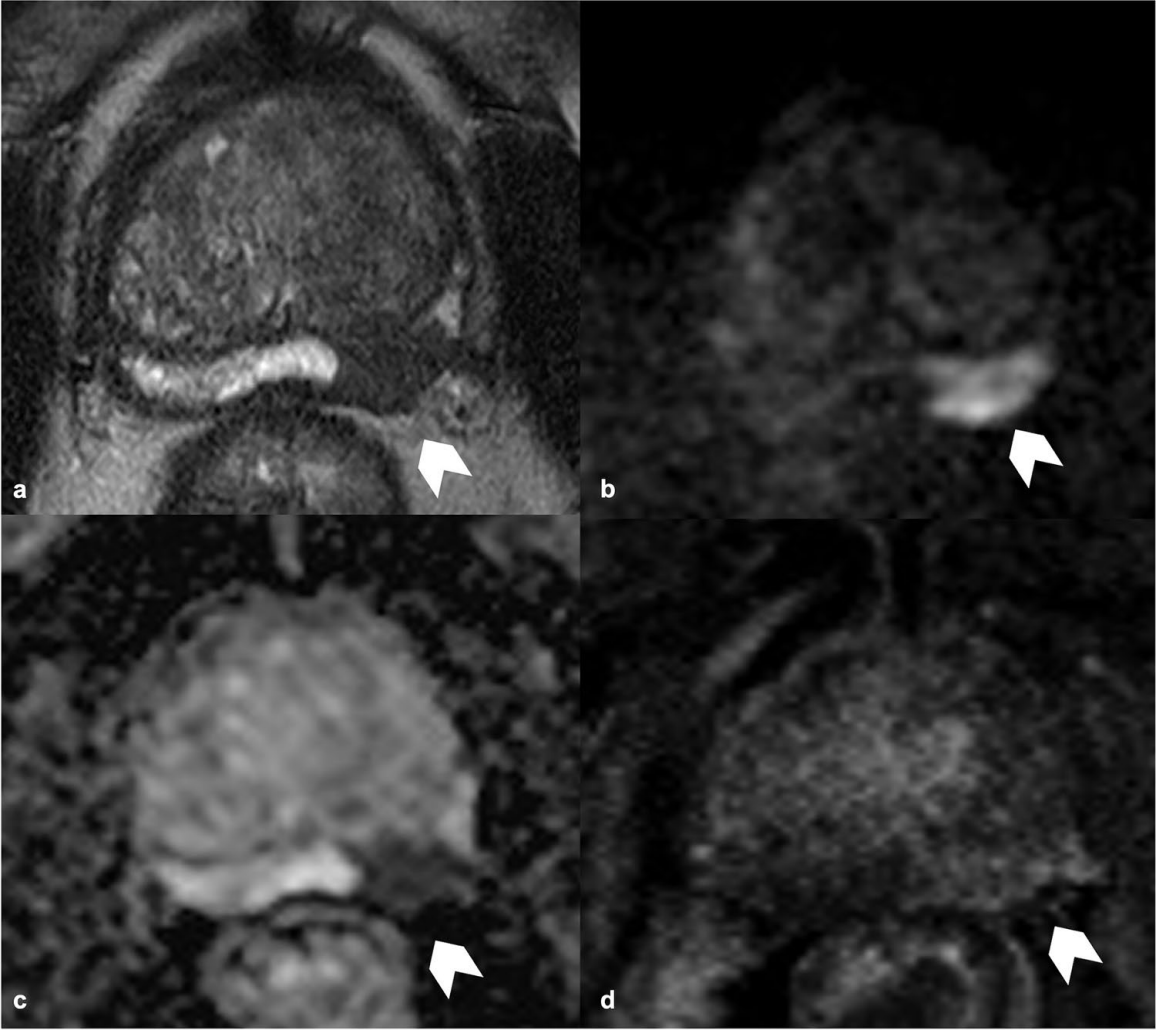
a T2WI on axial plane shows an alteration on the mid left posterior-lateral peripheral zone (white arrow head); b, c DWI (b = 2000) and ADC map show a focal and marked  hyperintensity on high b value and hypointensity on ADC map on the left peripheral zone lesion (white arrow head); d DCE shows an early and focal enhancement of the lesion. All the sequences demonstrate a lesion contact with the capsule measuring about 15 mm. T2WI, T2 weighted imaging; DWI, Diffusion Weighted Imaging; ADC, Apparent Diffusion Coefficient; DCE, dynamic contrast enhancement
